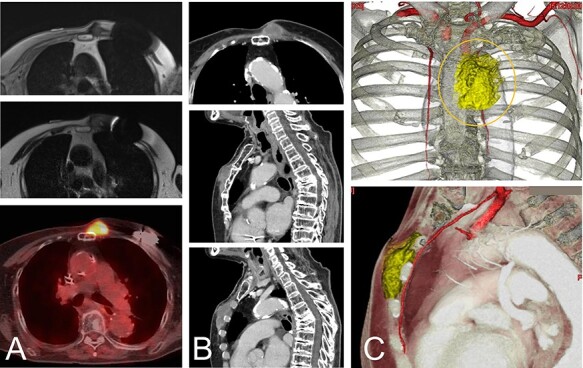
An 82-year-old woman with a malignant spindle cell sarcoma in the superficial chest wall. The MRI shows a lesion involving the anterior sternum on the left side with low-to-intermediate signal intensity on T1- (A-top) and T2-weighted images (A-middle) with a high SUVmax on FDG-PET (A-bottom). CT with contrast medium depicted the lesion (B). The lesion involved not only the anterior sternum (B-top and -middle), but also the intercostal space (B-bottom). The cut line (orange line) is drawn with a yellow line. The internal thoracic artery runs under the tumor and the vessels were resected together (C).
